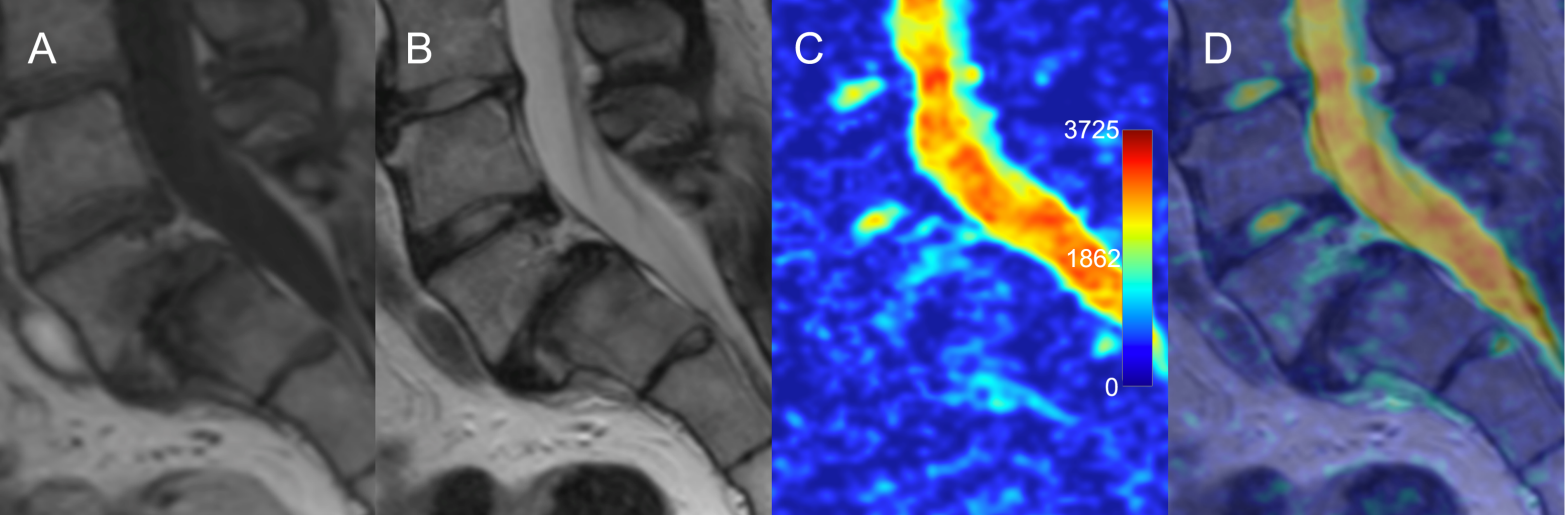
Modic type 1 changes in the setting of degenerative spondylolisthesis. (A) T1-WI shows hypointense signal; (B) T2-WI shows hyperintense signal; (C) ADC map shows hyperintense signal; (D) overlay of ADC map over T2 image depicts anatomical location of the regions with increased diffusivity corresponding to the margin of Modic 1 changes. ADC, apparent diffusion coefficient; T2-WI, T2-weighted image.
